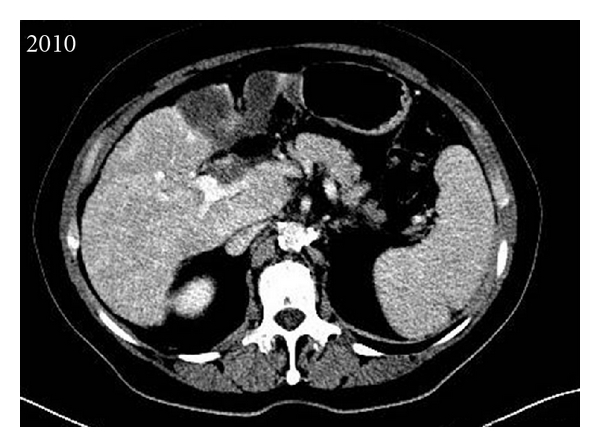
Case report: follow-up CT scan 4 months after injection of 5 GBq of in the central branch: major response.
